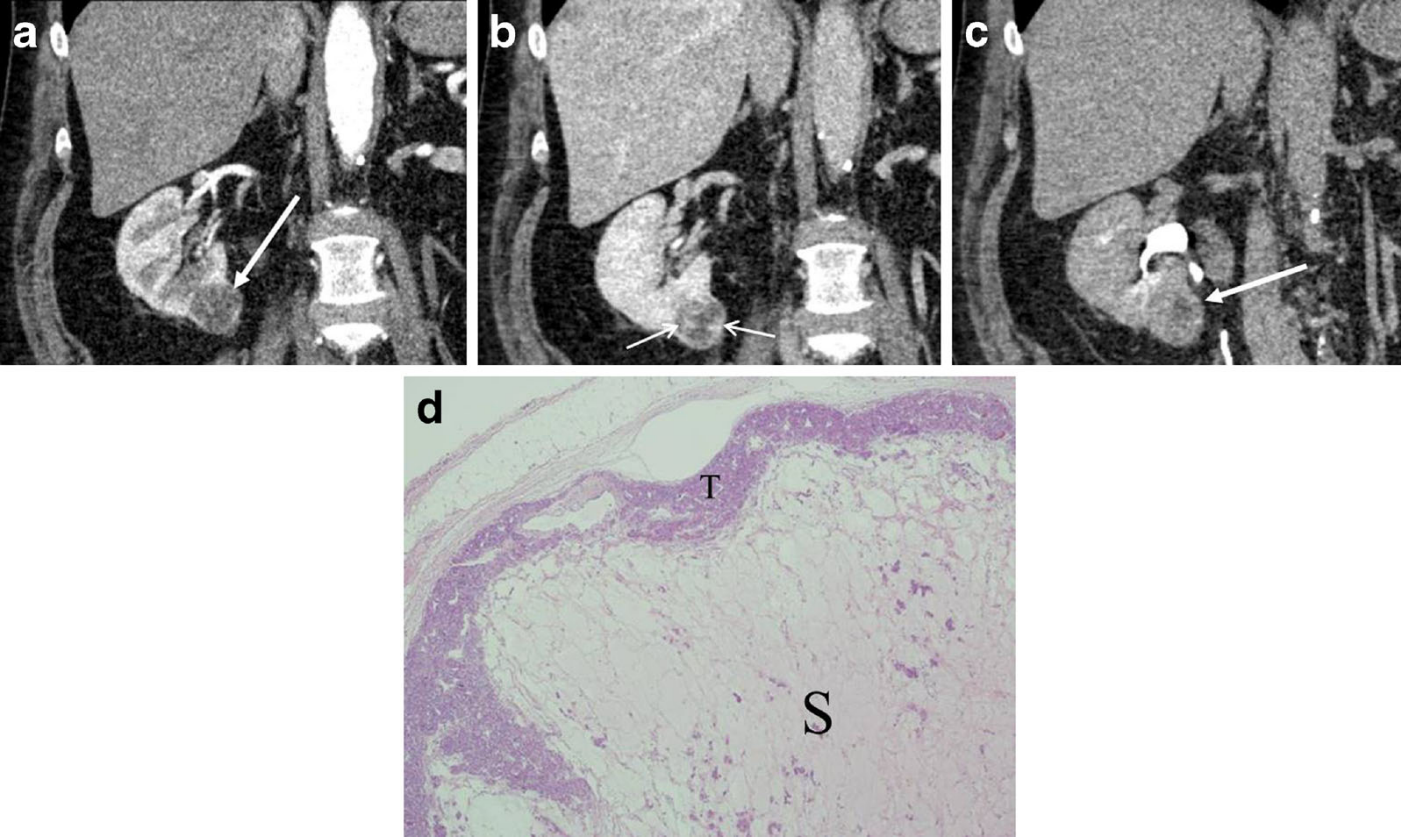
A 56-year-old man with right renal oncocytoma demonstrating hypovascularity and gradual enhancement. a Coronal reformatted image of the corticomedullary phase of a CE-CT shows a low attenuation mass in the lower right renal pole (*arrow*). b The nephrographic phase demonstrates patchy peripheral enhancement (*small arrows*). c The delayed phase shows gradual enhancement with a small residual area of low attenuation (*arrow*). d (Low magnification) Oncocytoma, a peripheral rim of tumour cells (*T*) surrounds abundant oedematous stroma (*S*)
